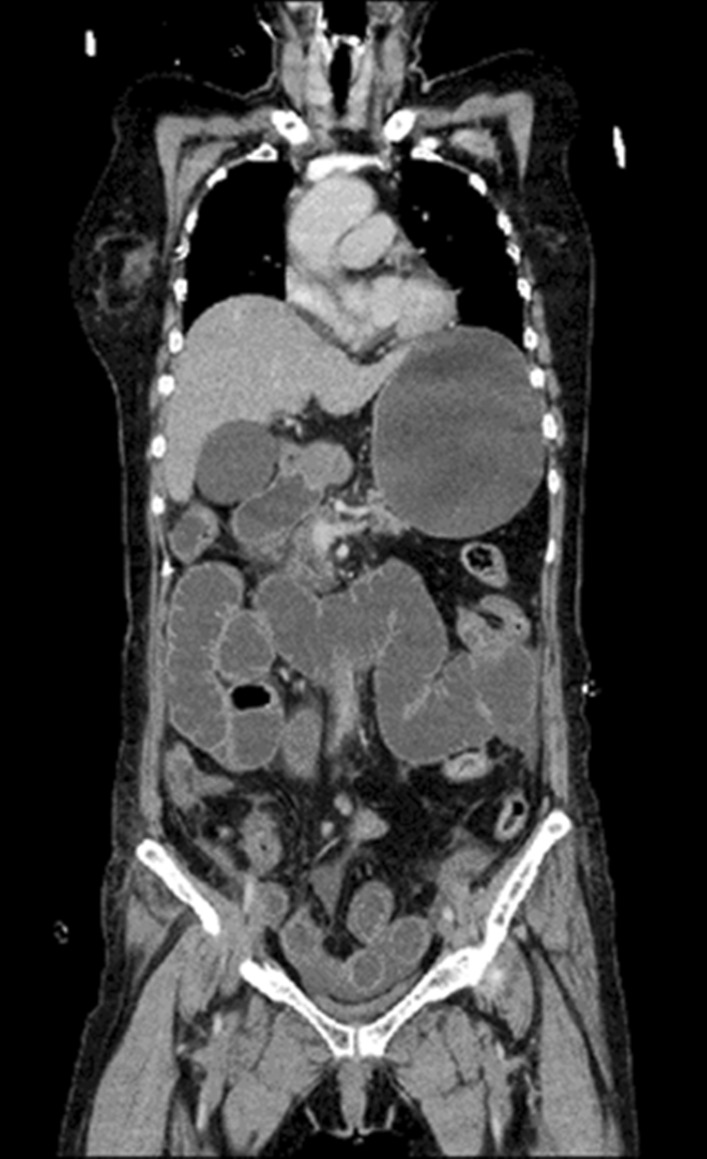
CT scan demonstrating remarkable intestinal dilation and thickening of the intestinal wall on the 5th day after the first chemotherapeutic cycle
